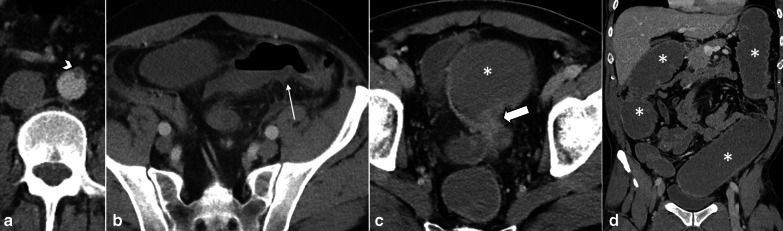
A 48 y/o male presented abdominal distention and diffuse pain. CT images demonstrate complete thrombotic occlusion of IMA (arrowhead) at its origin (a). Segmental thickening of sigmoid colon is noted in b with hypo enhancement, compatible with watershed ischemia (thin arrow). c 3 months later, patient returns with fibrotic stricture (thick arrow) of the distal sigmoid colon with upstream colonic dilatation and obstruction in d (asterisk)
